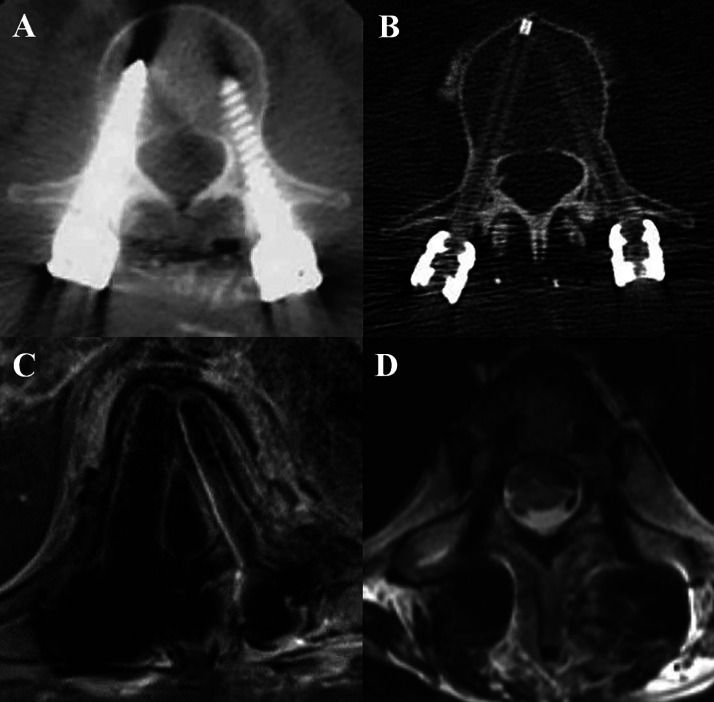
Comparison of image quality between titanium and Carbon/PEEK implant. (A) Axial CT image of a titanium implant. (B) CT image of a hybrid Carbon/PEEK implant. (C) Axial MR image of a titanium implant. (D) MR image of a hybrid Carbon/PEEK implant. *Abbreviations*: Carbon/PEEK = carbon fiber-reinforced polyetheretherketone; CT = computed tomography; MR = magnetic resonance.
